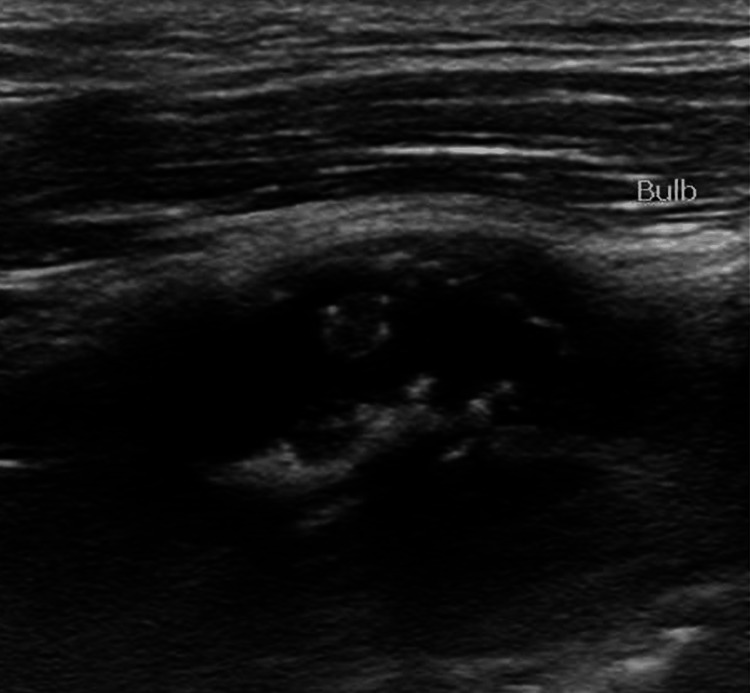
Carotid artery ultrasound (long-axis view) of the left internal carotid artery. Near the left common carotid artery bulb, there is a mobile atheroma.
